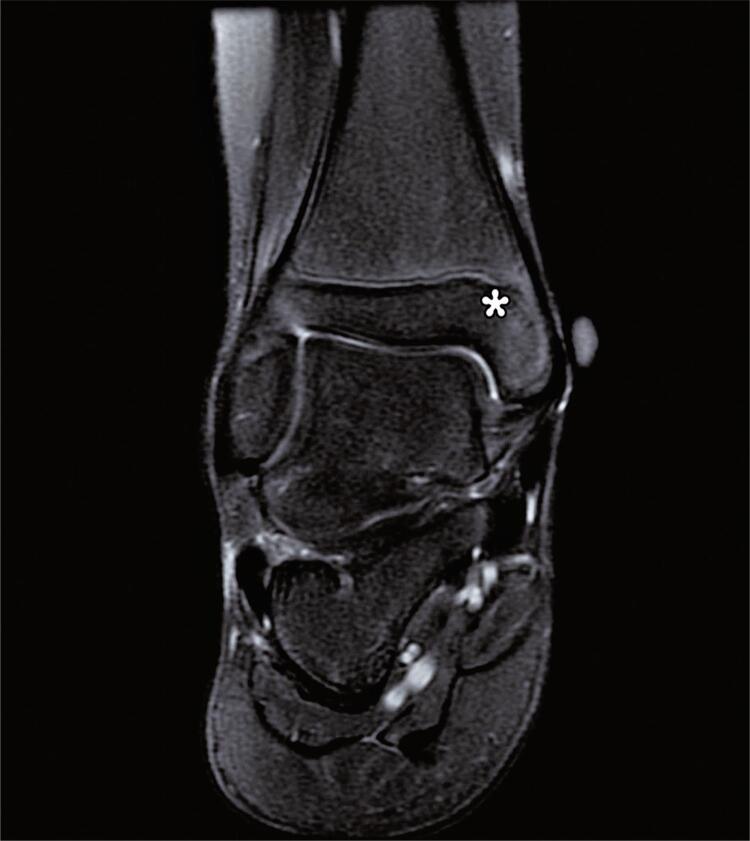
Coronal T2-weighted fat-suppressed magnetic resonance image shows bone bruise at the medial malleolus (star)
